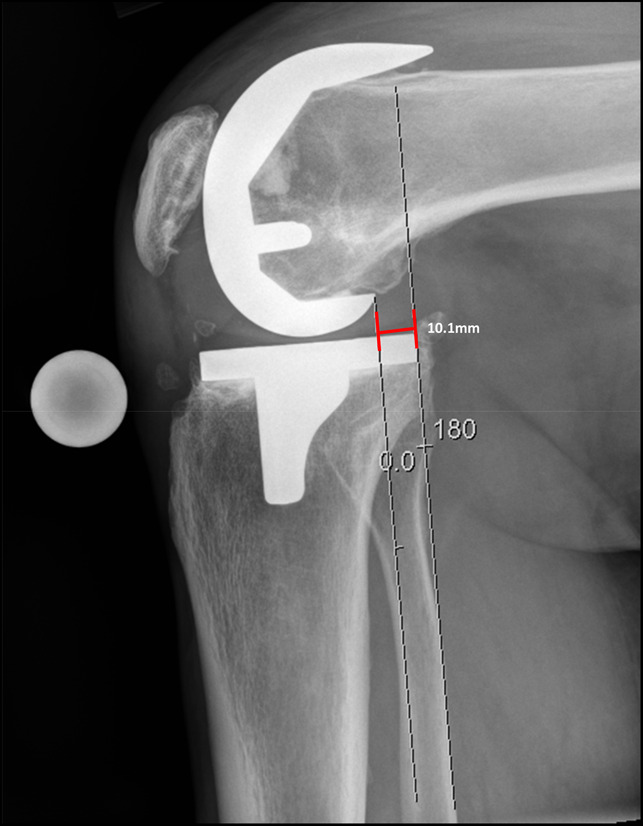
Prestress lateral radiograph of the knee demonstrating 10.1 mm of space between the posterior femoral condyle and the posterior aspect of the tibial baseplate.
